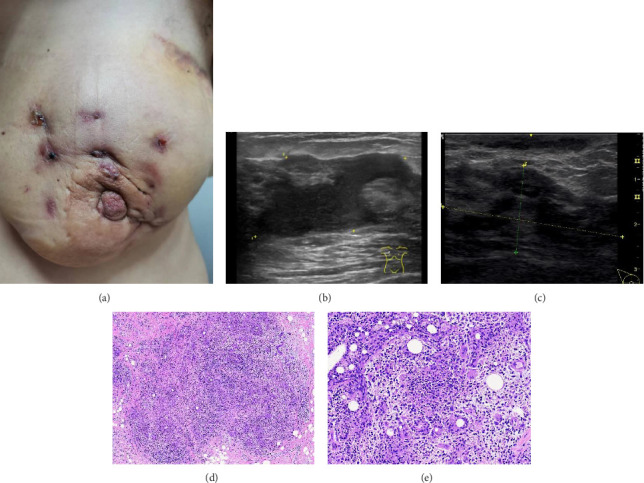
Diagnosis of severe IGM. (a) The breast appearance of a typical case with severe IGM. (b) Breast ultrasonography of a large lesion with abscess formation. (c) Breast ultrasonography of fused multiple lesions. (d) Pathology magnification ×100. (e) Pathology magnification ×400.
